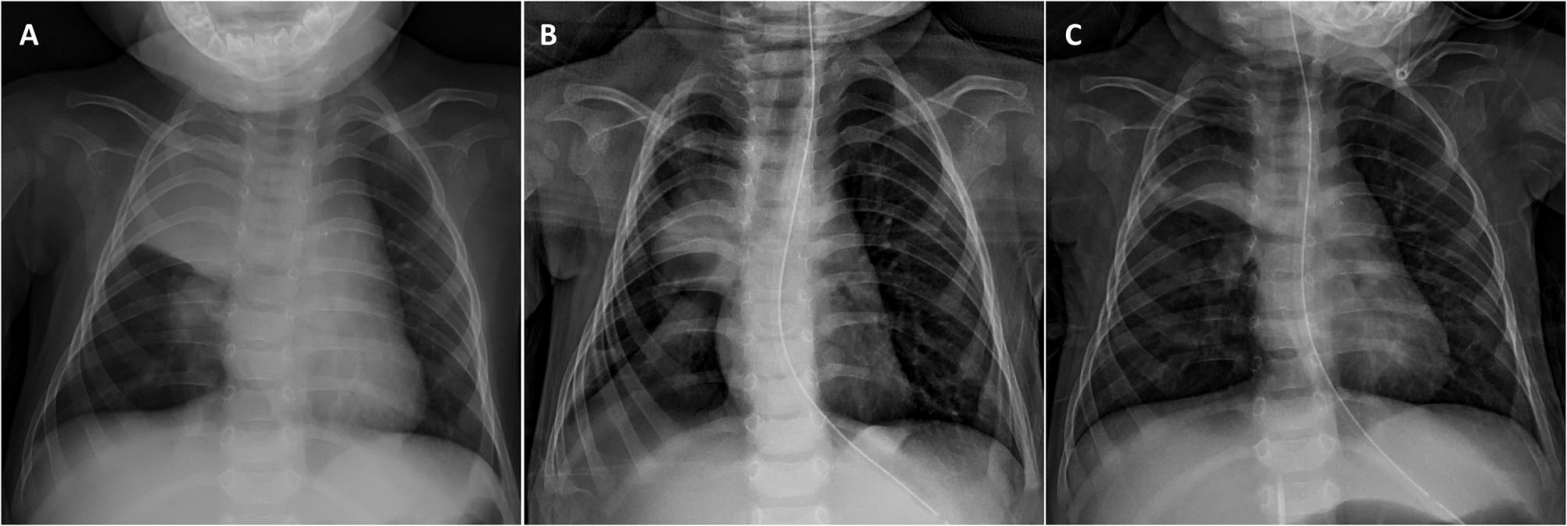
Resolution of right upper lobe opacification in an infant with severe type 1 spinal muscular atrophy (SMA) after combining NIV and MI-E. (A) Chest X-ray on admission showing right lung pneumonia with significant atelectasis complicated by copious secretions. (B) A significant improvement was found after 2-days treatment, with a resolution of atelectasis. (C) A progressive improvement of the pneumonic patch was observed on day 7 when discharged from PICU.
